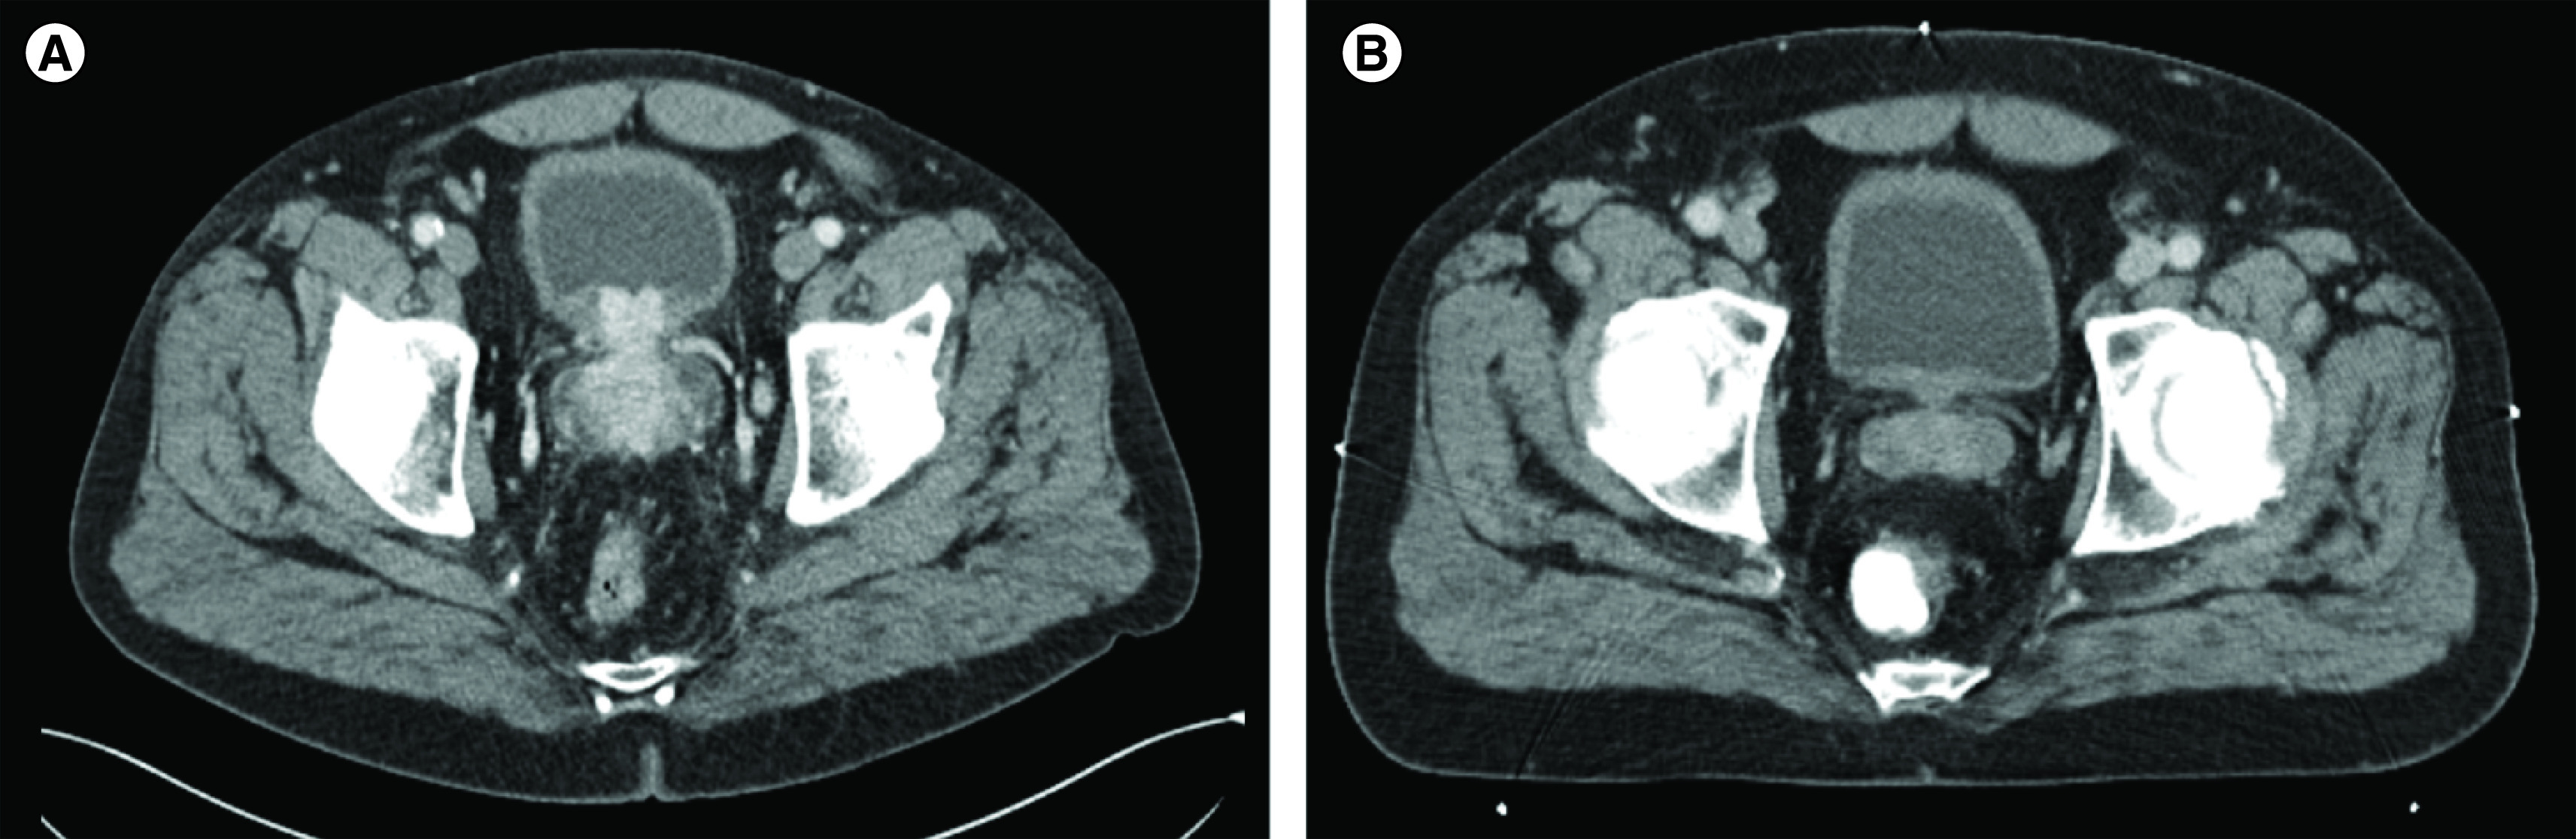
Radiological evolution on androgen deprivation therapy. (A) Initial abdominal CT showing locally advanced prostate cancer with bladder wall infiltration. (B) Abdominal CT after 12 months of androgen deprivation therapy, with a regression of the tumor and of the bladder infiltration. No peritoneal involvement was detected on the two CTs. CT: Computed tomography.
